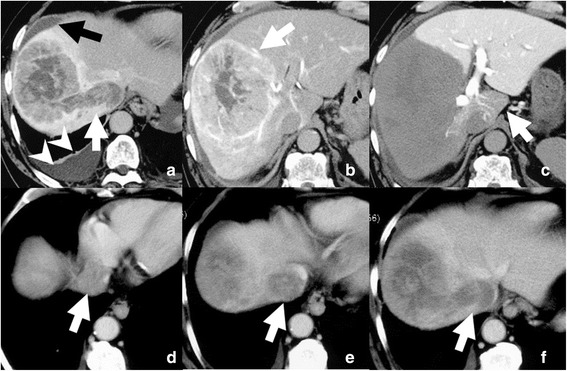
a CT during hepatic arteriography shows a heterogenous hypervascular tumor and venous tumor thrombus, corresponding to the right hepatic vein, extending into the IVC (*white arrow*). Ascites (*black arrow*) and pleural effusion (*white arrowheads*) is also documented. b CT during hepatic arteriography shows a heterogenous hypervascular tumor measuring 11.5 cm in diameter (*white arrow*). c CT during arterial portography shows a tumor defect and hypoperfused caudate lobe (*white arrow*); nevertheless, the caudate branch of the portal vein is patent. This implies congestion of the caudate lobe due to the IVC tumor thrombus. d–f The tip of the venous tumor thrombus extends into the right atrium (*white arrow*)
